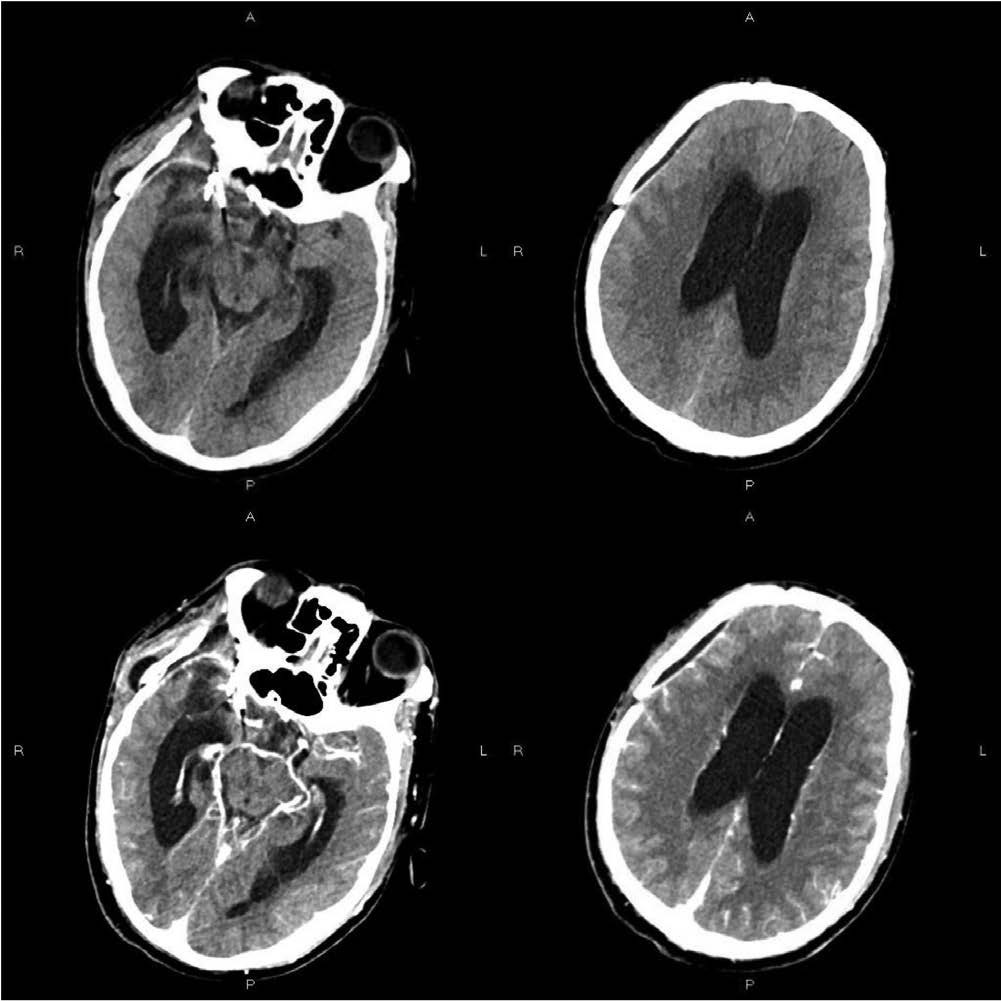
Enhanced CT scan on October 21, showed a small amount of subcutaneous effusion, subdural effusion, hydrocephalus and interstitial brain edema around bilateral lateral ventricles (A) CT scan on October 29, showed that the right temporal subcutaneous effusion and subdural effusion were less than that before. (B) Computed tomography.
